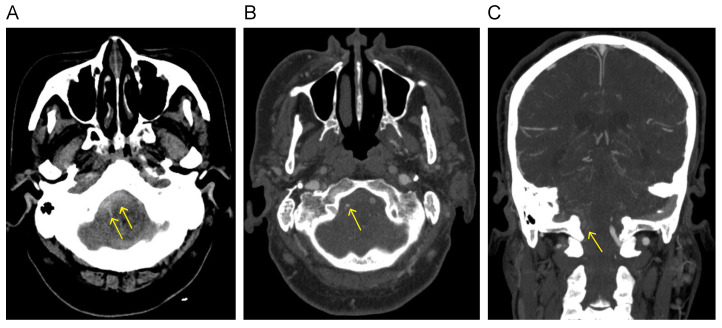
(A) Initial CT scan displays SAH (arrow) in the posterior fossa and around the midbrain. CT angiography reveals a tiny suspected aneurysm (arrow) at the right cerebellomedullary cistern in axial (B) and coronal views (C).
